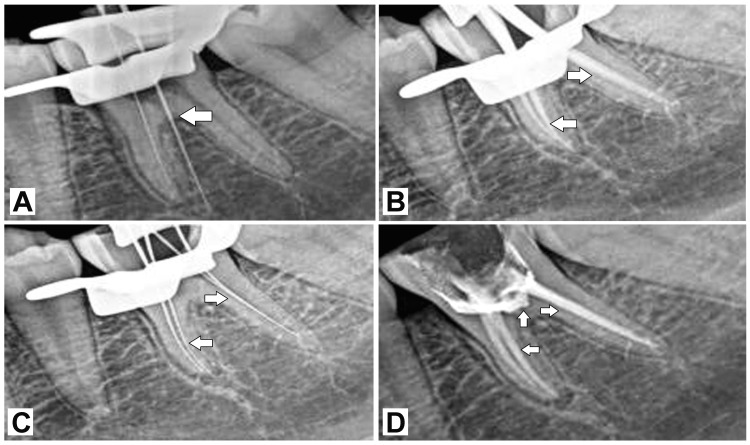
Radiographic insights of perforation and treatment insights: (A) radiograph showing perforation in furcation region with 46; (B) radiograph showing master cone selection with 46; (C) re-identified canals with 46; (D) obturated canals with gutta percha with 46 and perforation sealed with MTA with 46 Courtesy: Shreeya Panchal
